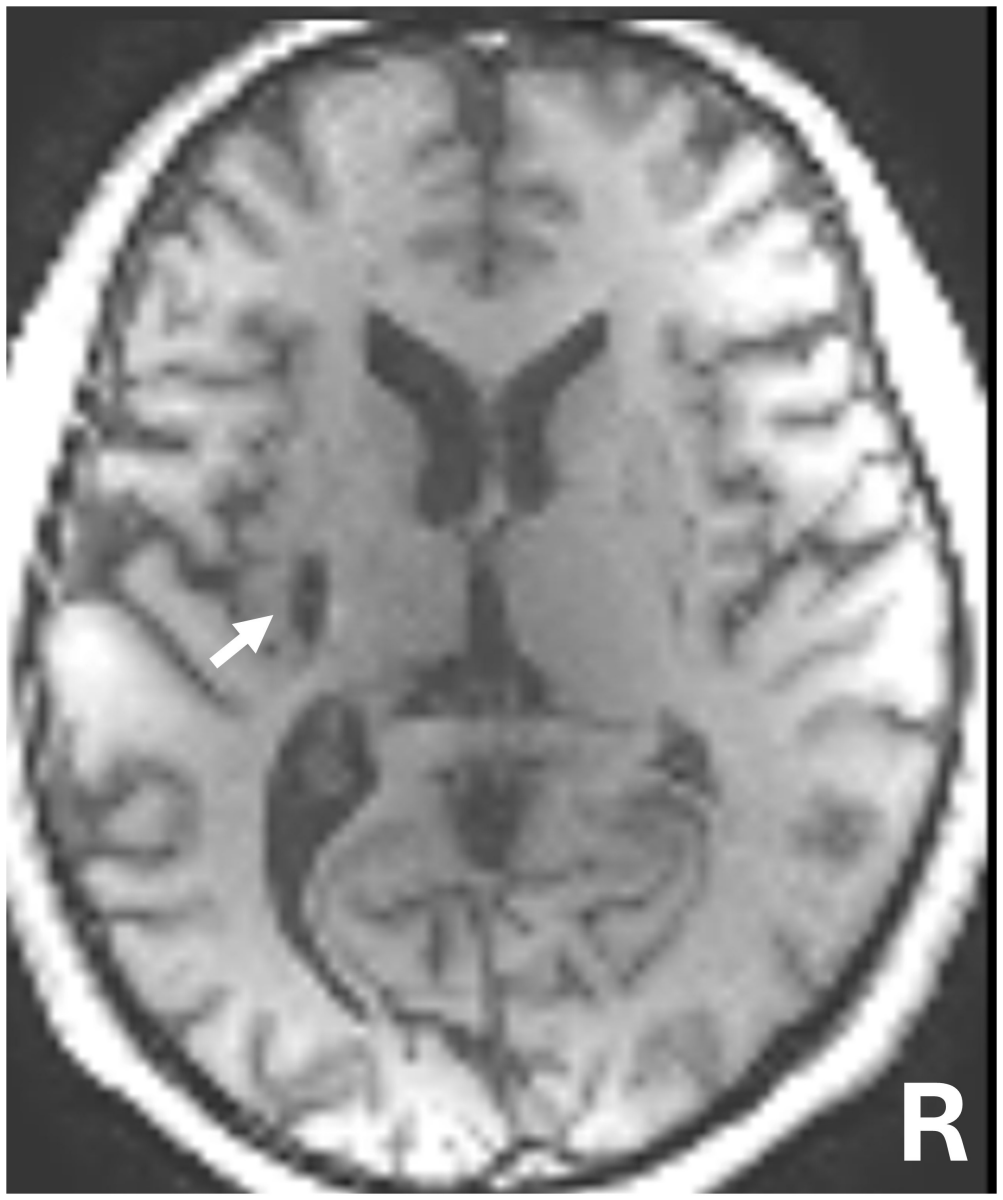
T1-weighted magnetic resonance imaging (MRI) 5 years following brain hemorrhage onset. The white arrow indicates the left putamen lesion.
